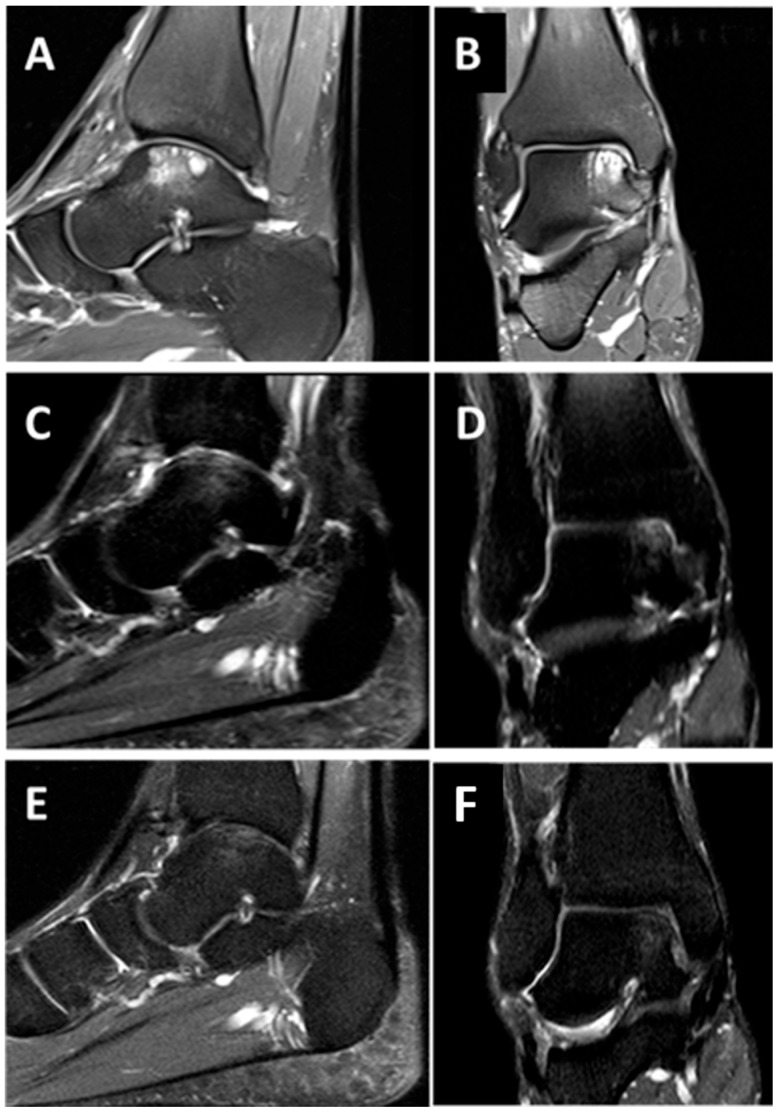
Ankle MRI in a patient with an osteochondral lesion of the talus (OLT): preoperative: (A) sagittal view; (B) coronal view; 6 months postoperatively: (C) sagittal view; (D) coronal view; 12 months postoperatively: (E) sagittal view; (F) coronal view.
